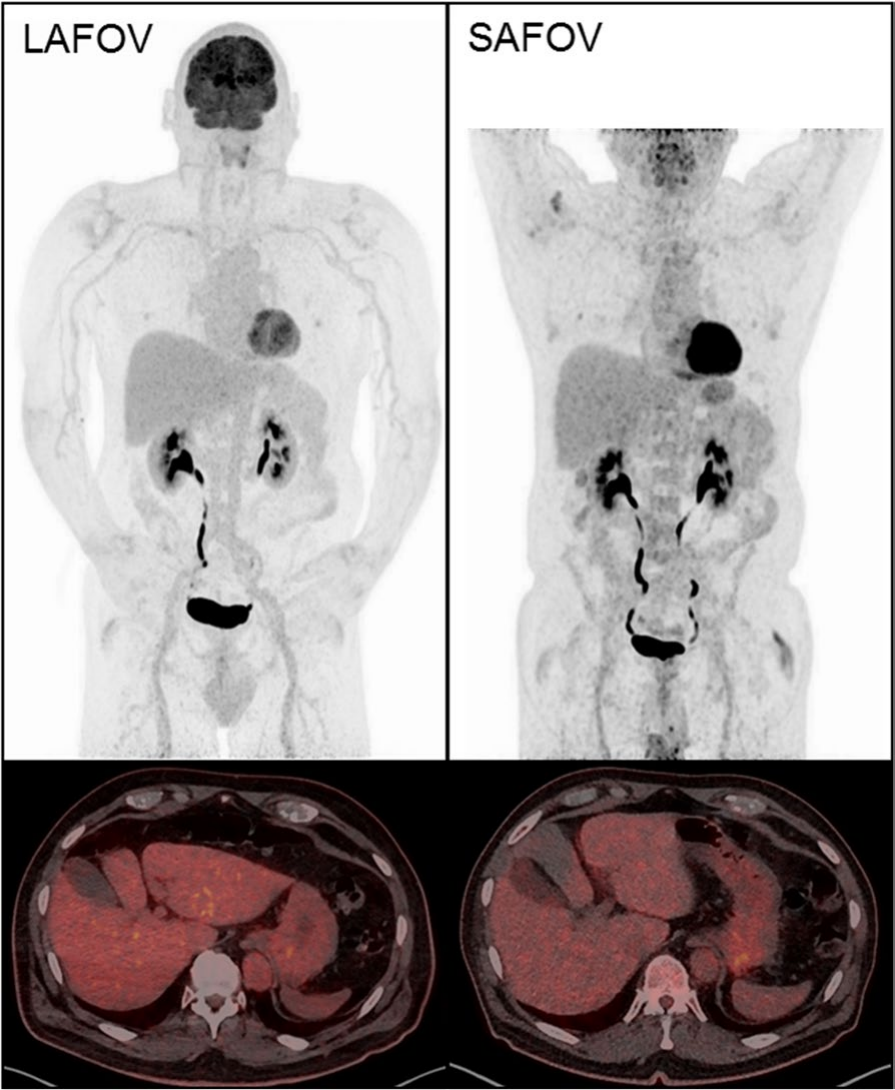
Indicative imaging quality for a low dose PET/CT acquisition on a LAFOV with 20 MBq of 2-[^18^F]FDG (left) and a full dose with 340 MBq on an analogue SAFOV system (right). The scan times for both are equivalent at 20 min. Maximal intensity projections (MIP) and co-registered PET/CT axial slices (bottom) for the same patient are shown
